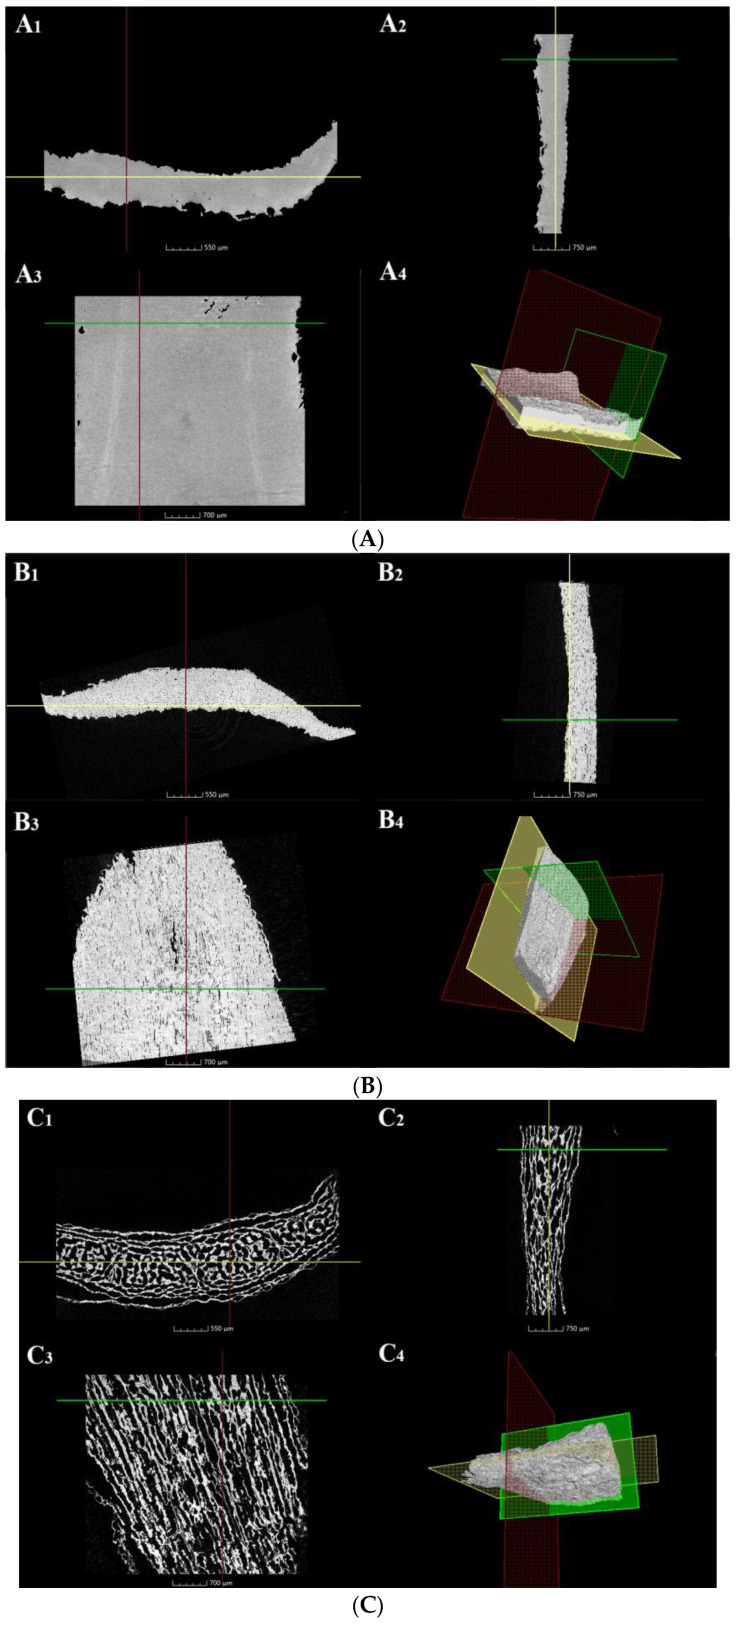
Micro X-ray computed tomography (CT) images of dried lily scales. (A–C) are the CT images for HAD, MVD, and VFD, respectively; (A_4_,B_4_,C_4_) are the three-dimensional CT images; (A_1_–A_3_), (B_1_–B_3_), and (C_1_–C_3_) are CT images of perpendicular slice; those colored planes or lines at images indicate each slice location.
